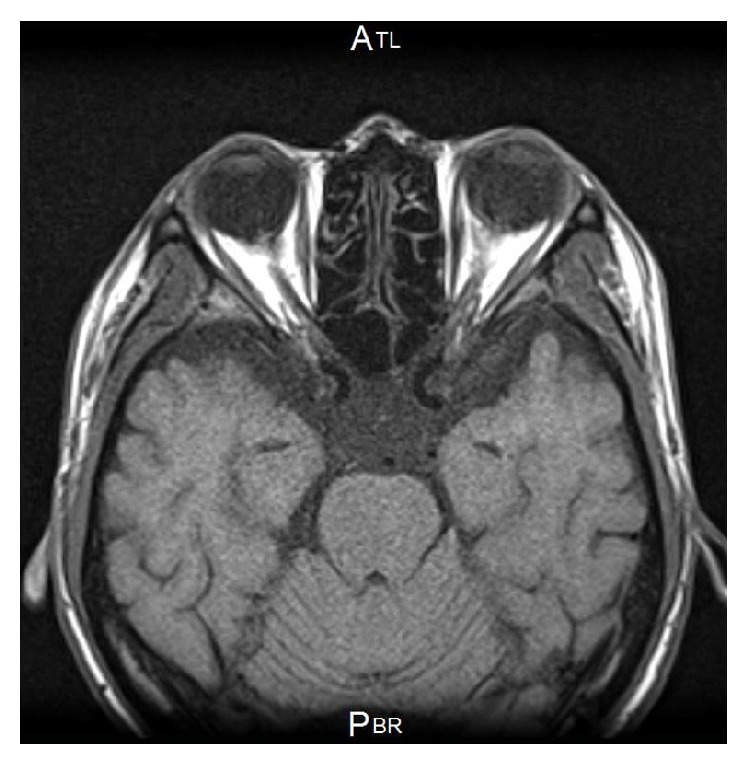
MRI findings in patient with hematopoietic malignancy. The MR images did not detect any abnormalities.
